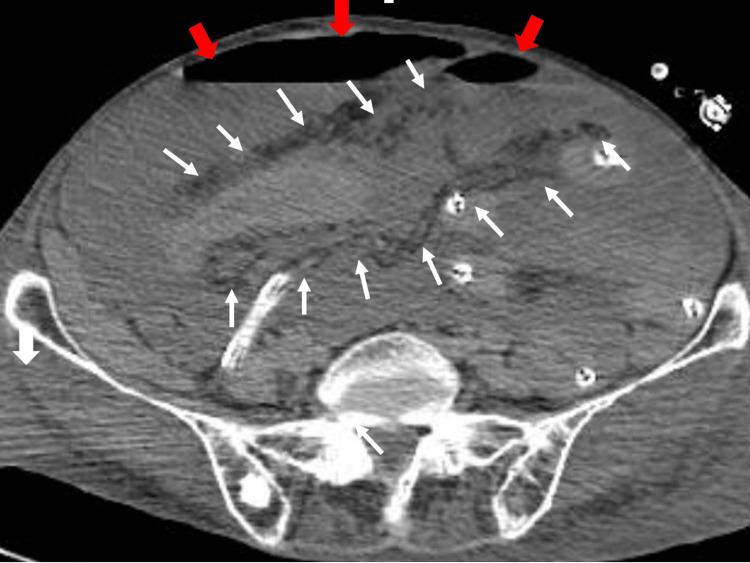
Pelvic computed tomography showing free air just below the abdominal wall (red arrows) and between the small intestine (white arrows)
